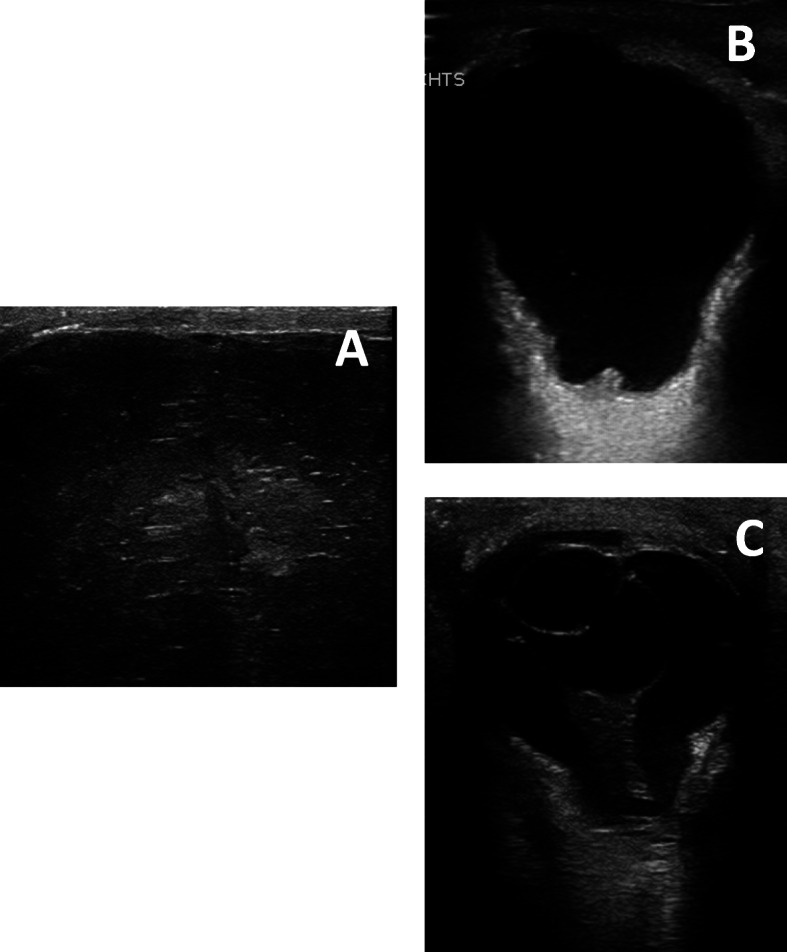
Postnatal ultrasound of the brain / eyes; A lissencephaly with polymicrogyria; b staphyloma; c persistent hypoplastic primary vitreous body
